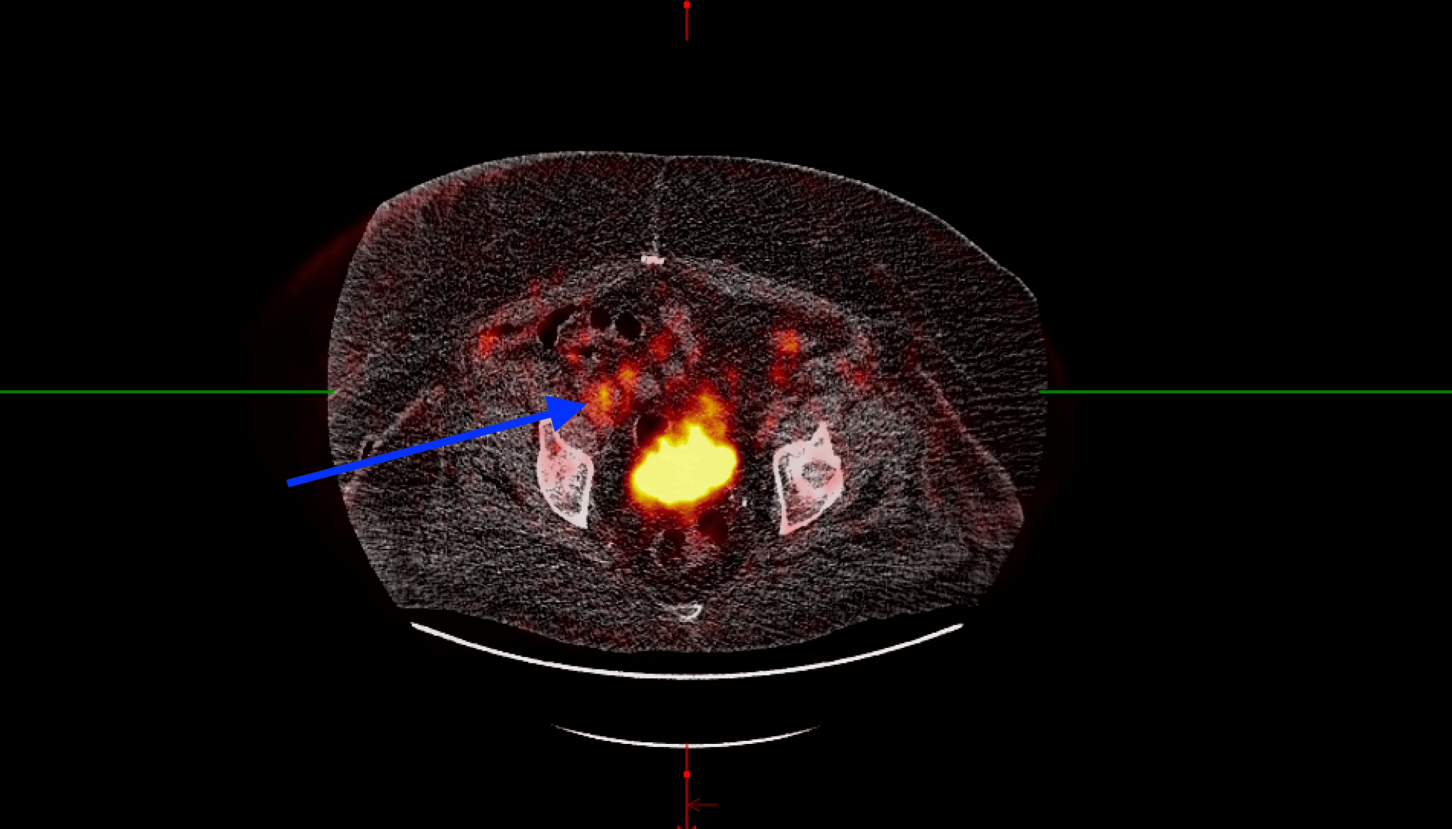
Positron emission tomography (PET) scan with an arrow showing a new fluorodeoxyglucose (FDG)-avid lymph node within the right inguinal region measuring 1.9 x 3.7 x 2.4 cm with a maximum standardized uptake value (SUV) of 5.10, indicating increased metabolic activity associated with cancerous growths, but can also be seen in inflammation or infection.
